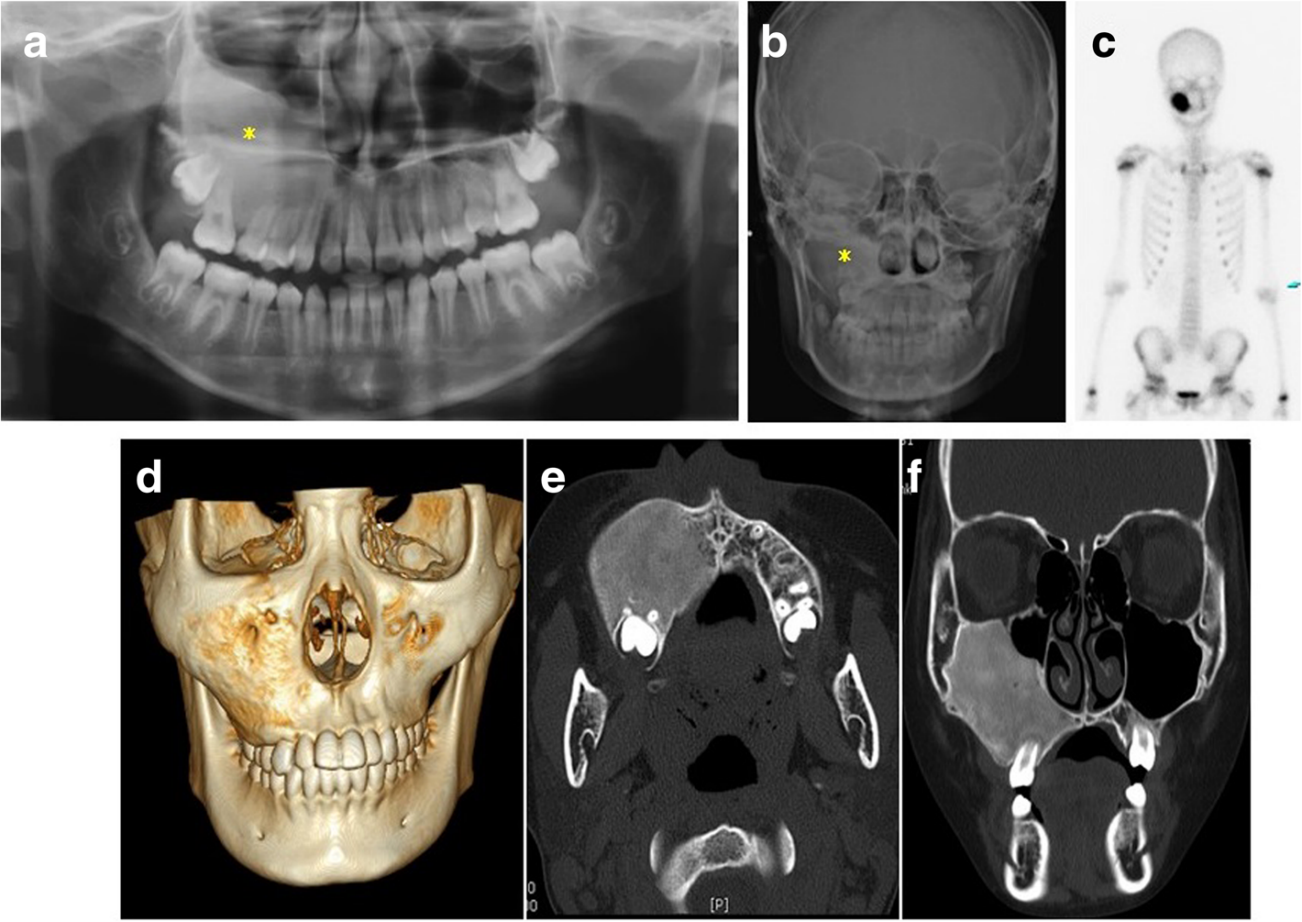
Preoperative radiographic images. a Panoramic view showed a high bone density on the right maxilla and zygoma and obliteration of the right maxillary sinus (asterisk indicates the fibrous dysplasia lesions). Additionally, the development of tooth germ was observed in the lesion. b Posteroanterior (PA) cephalometric view also showed an increased bone density on the right maxillary area and an asymmetric facial contour. c Bone scan showed an irregularly shaped hot uptake in the right maxilla, and no abnormally increased uptake was observed in any other sites. d Preoperative 3D CT image showed the enlarged right maxilla and zygomatic body. e, f Computed tomography (CT) showed 4.5 × 4 × 4.5 cm expansile ground-glass opacity lesion widely involving the right maxillary sinus, right maxillary alveolar process, and hard palate
